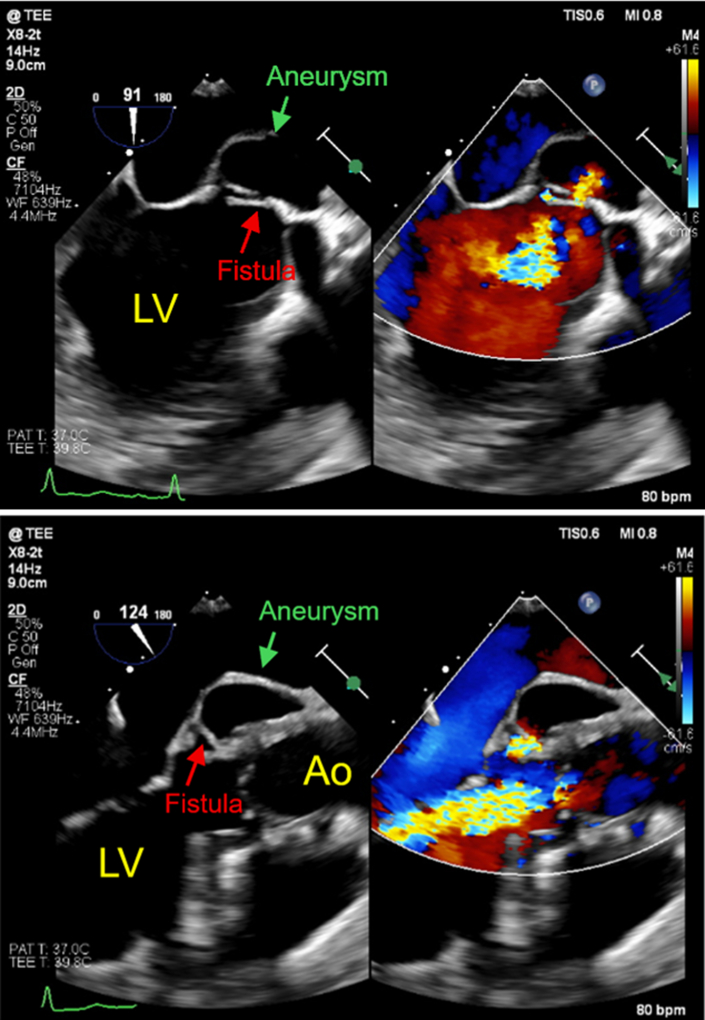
Preoperative Transesophageal Echocardiography Transesophageal echocardiography revealed a systolic jet from the aorta entering the NCC-adjacent aneurysm, which communicated with both the LVOT-side and NCC-side aneurysms via separate 3-mm-diameter channels. During diastole, a jet from the LCC-side aneurysm into the LVOT was observed. Moderate AR was noted.
